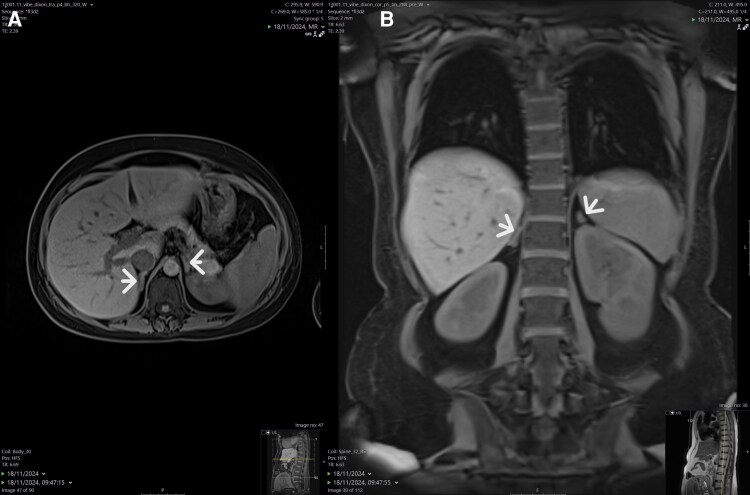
T1-weighted abdominal magnetic resonance imaging at the level of the adrenal glands (arrows): A, axial plane; B, coronal plane.
